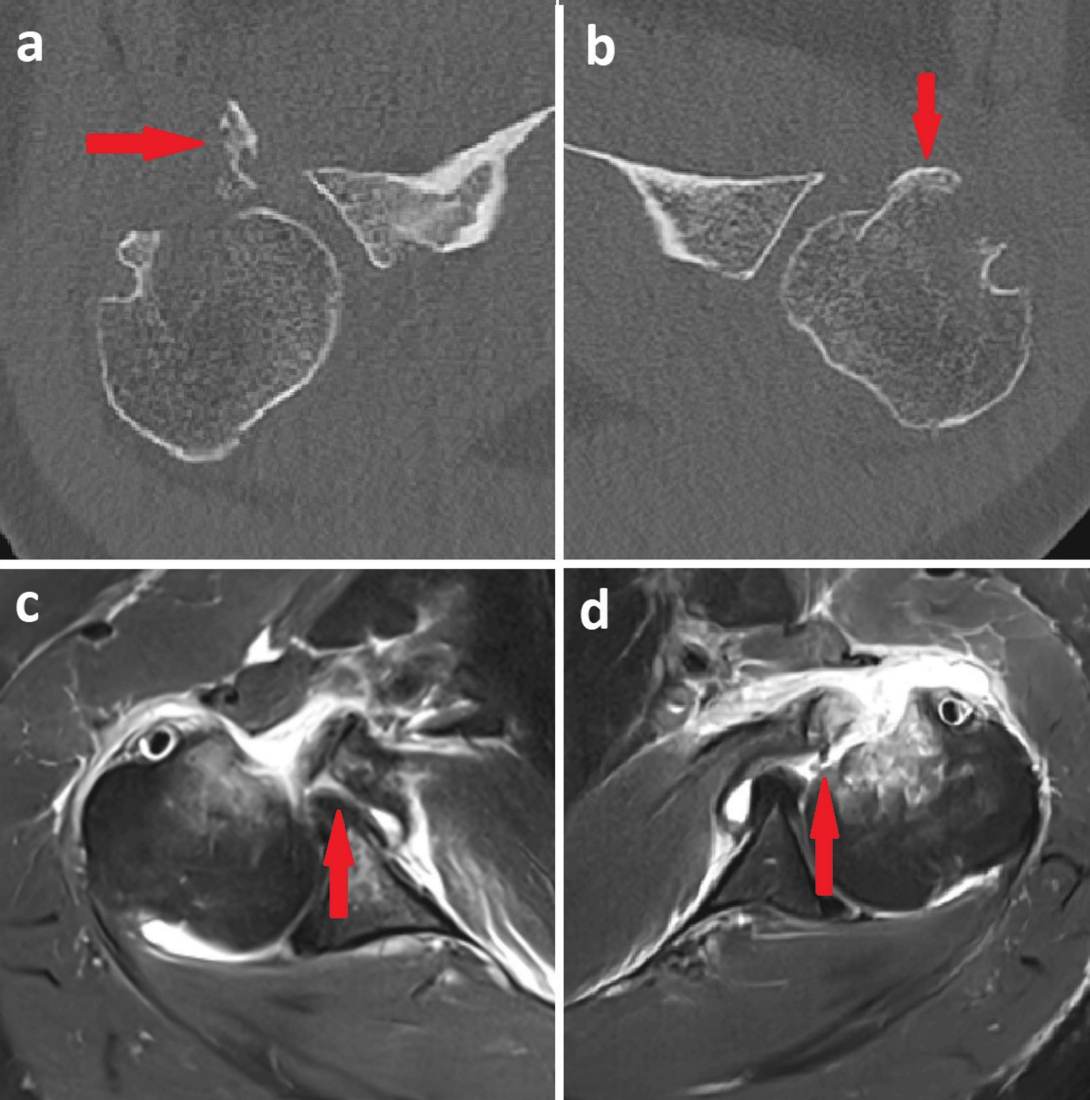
CT (a,b) and MRI (c,d) of the right and left shoulders showing displaced lesser tuberosity fragments (>5 mm) with the subscapularis tendon attached (red arrows) and diffuse osteopenic bone.
